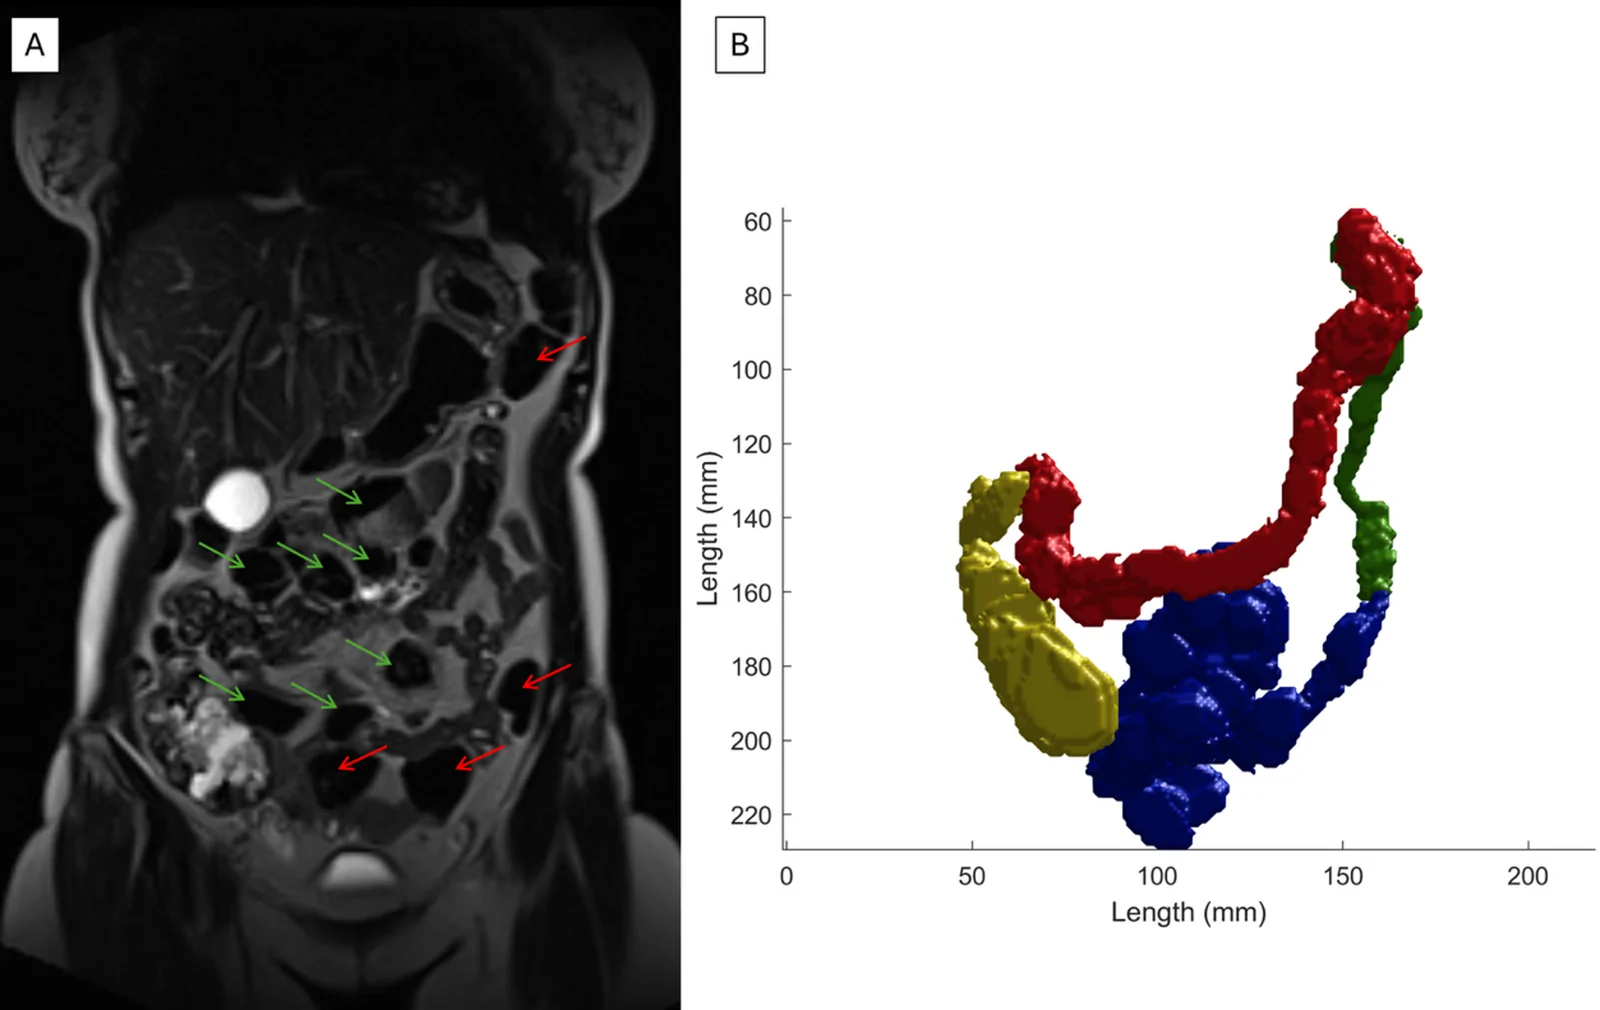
A Coronal T2-weighted MRI of the abdomen showing gas in the small bowel (green arrows) and in the colon (red arrows). B 3D-model of the segmented colon volume. The ascending colon is yellow, the transverse colon is red, the descending colon is green, and the sigmoid colon/rectum is blue
